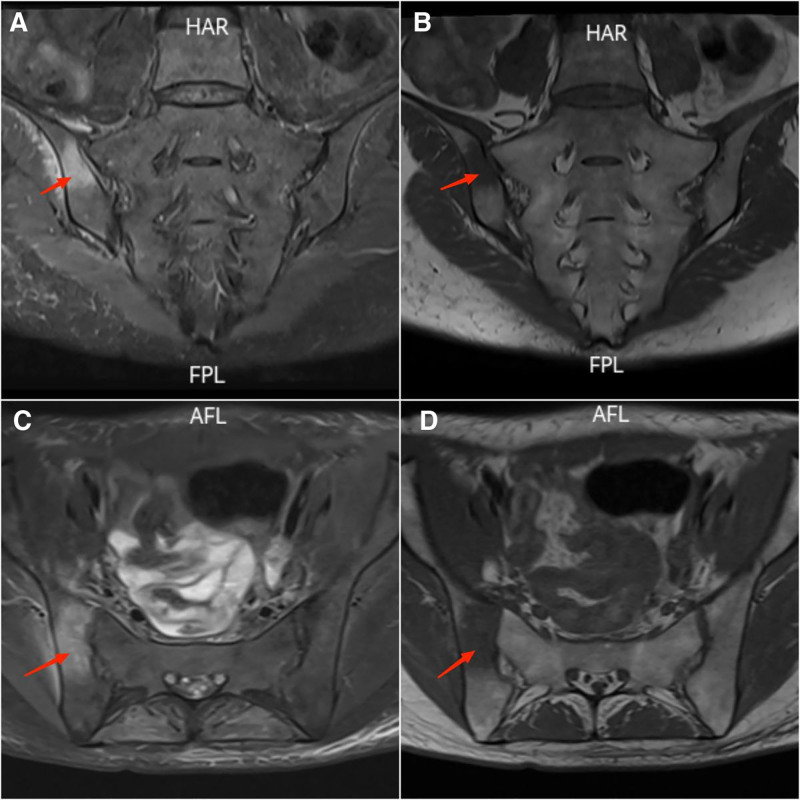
Follow-up MRI of the sacroiliac joints after 4 weeks of antibiotic therapy. (A and B) PD-weighted images demonstrate residual high signal intensity (arrow) on the iliac side of the right sacroiliac joint, indicating persistent bone marrow edema; however, the extent and intensity are significantly reduced compared to admission. The right joint space remains slightly narrowed. (C and D) Corresponding T1-weighted images show patchy low signal intensity (arrow) in the same region. Note the resolution of gluteal and soft-tissue swelling. The left sacroiliac joint remains normal in alignment, signal, and joint space. MRI = magnetic resonance imaging.
